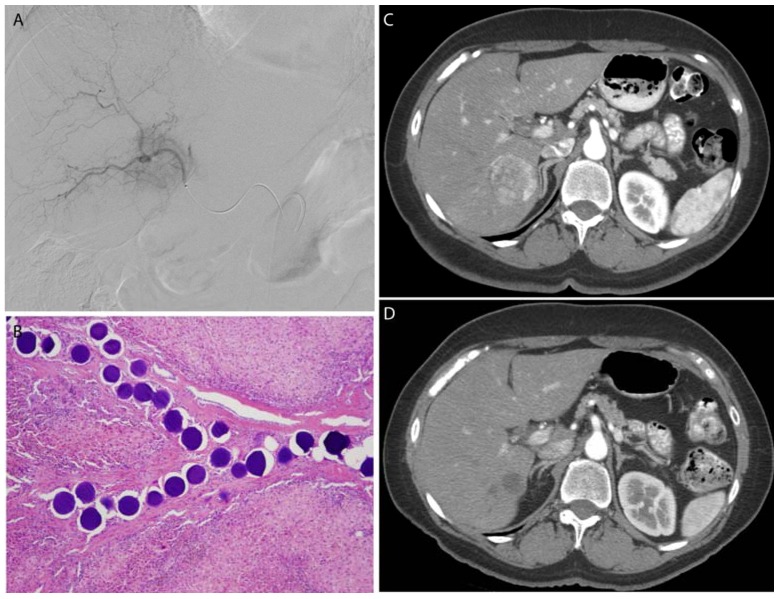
A breast cancer metastasis to the liver was embolized with 100–300 μm, doxorubicin-eluting microspheres to reduce tumor size and vascularity prior to surgical resection. Selective catheterization (A) of the tumor was performed, with delivery of the microspheres within the blood vessels of the tumor (B). Post-procedure CT (D) demonstrates complete response of the tumor as indicated by fibrosis alone without any evidence of residual tumor compared to the pre-procedure CT (C).
